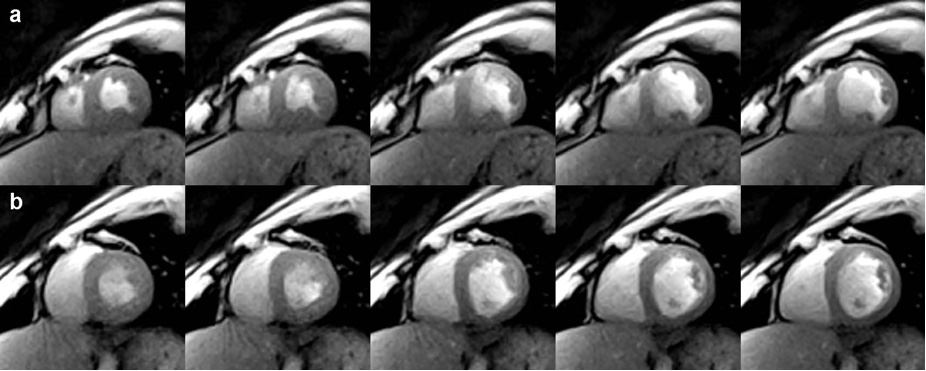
Interleaved dual-slice real-time radial FLASH CMR. T1-weighted images (spoiled multi-slice FLASH, TR = 4.0 ms for two sections, TE = 2.0 ms, flip angle 10°, 11 spokes, 44 ms total acquisition time) of two simultaneously acquired short-axis views (a) in the apical segment and (b) 20 mm above in the mid-cavity segment (2.0 mm resolution, 8 mm section thickness). The images cover a post-systolic expansion phase by every second frame (88 ms apart). They were selected from respective real-time CMR movies reconstructed by nonlinear inversion and zoomed by a factor of 1.5.
